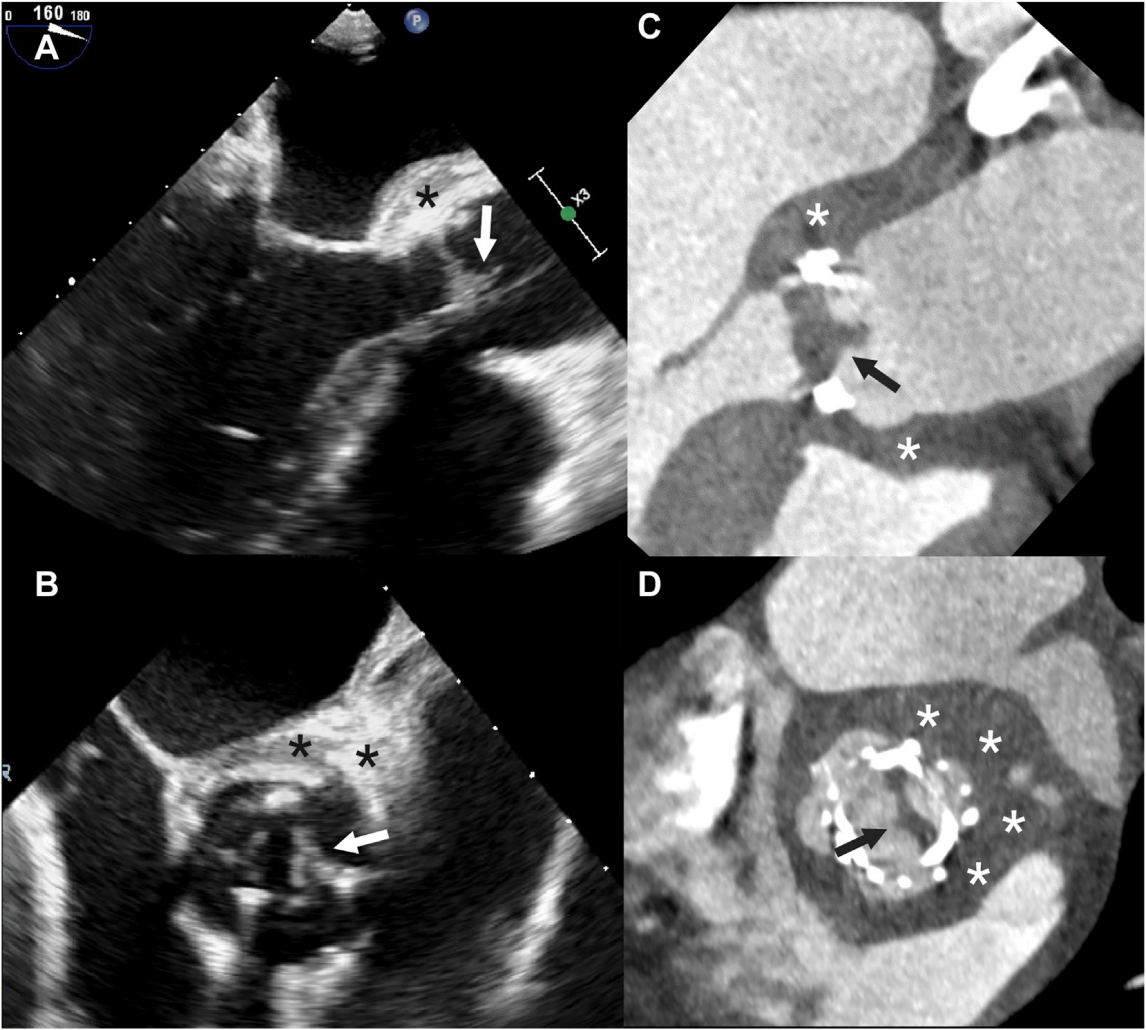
Thickened valve leaflets and vegetations in a patient with aortic biological prosthetic heart valve endocarditis. Thickened valve leaflets and vegetations on the valve are seen on both transesophageal echocardiography (A and B) and computed tomography (C and D). Also, notice the thickening of the aortic root (asterisks) indicating aortic root abscess formation.
